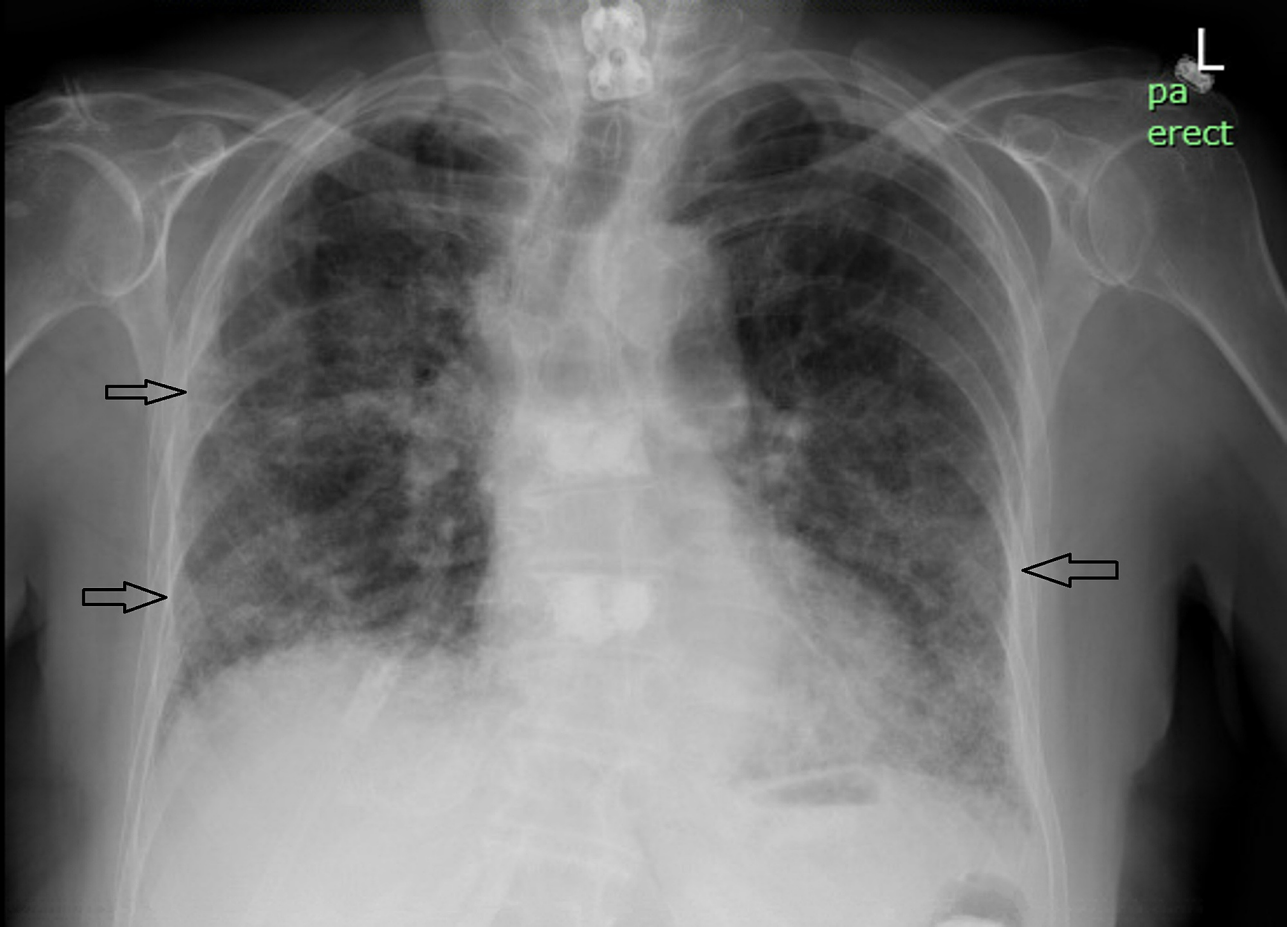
Chest X-ray. Arrows showing diffuse interstitial opacity
